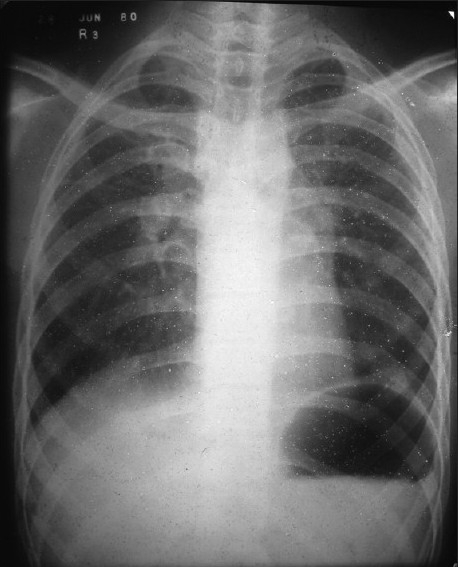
Chest X-ray (1980) showing bilateral hilar and left paratracheal lymphadenopathy with granular infiltrates in both upper lobes
